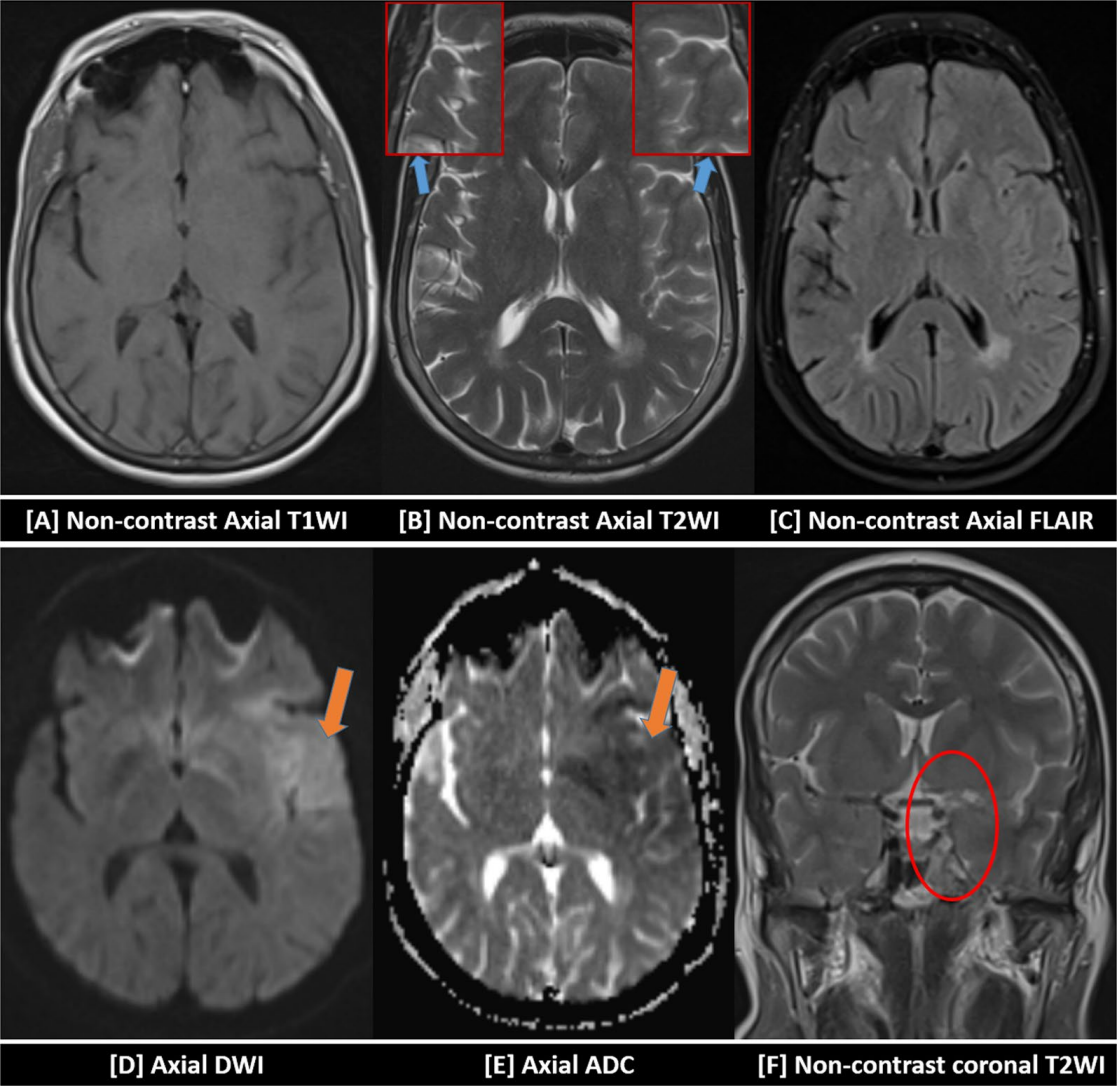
A-63 years-old female patient, with a history of cardiac disease, complained of disturbed level of consciousness and right hemiparesis two hours before visiting the emergency unit. No chest symptoms were reported. Urgent non-contrast brain CT was unremarkable on admission. Brain MRI was performed after two hours. Hypo-intense signal was noticed at the left temporal cortical and subcortical white matter in T1-WI [A]. A subtle cortical hyper-intense signal was barely noticed in T2-WI [B] and FLAIR-WI [C]. Positive diffusion restriction was noted in the form of a bright DWI signal [D] and a corresponding low ADC value in the ADC mapping [E] (orange arrows). Coronal T2-WI revealed non-visualization of the signal voids of the left ICA and MCA (red circle) [F] The initial diagnosis was a recent ischemic infarction along the left MCA territory
